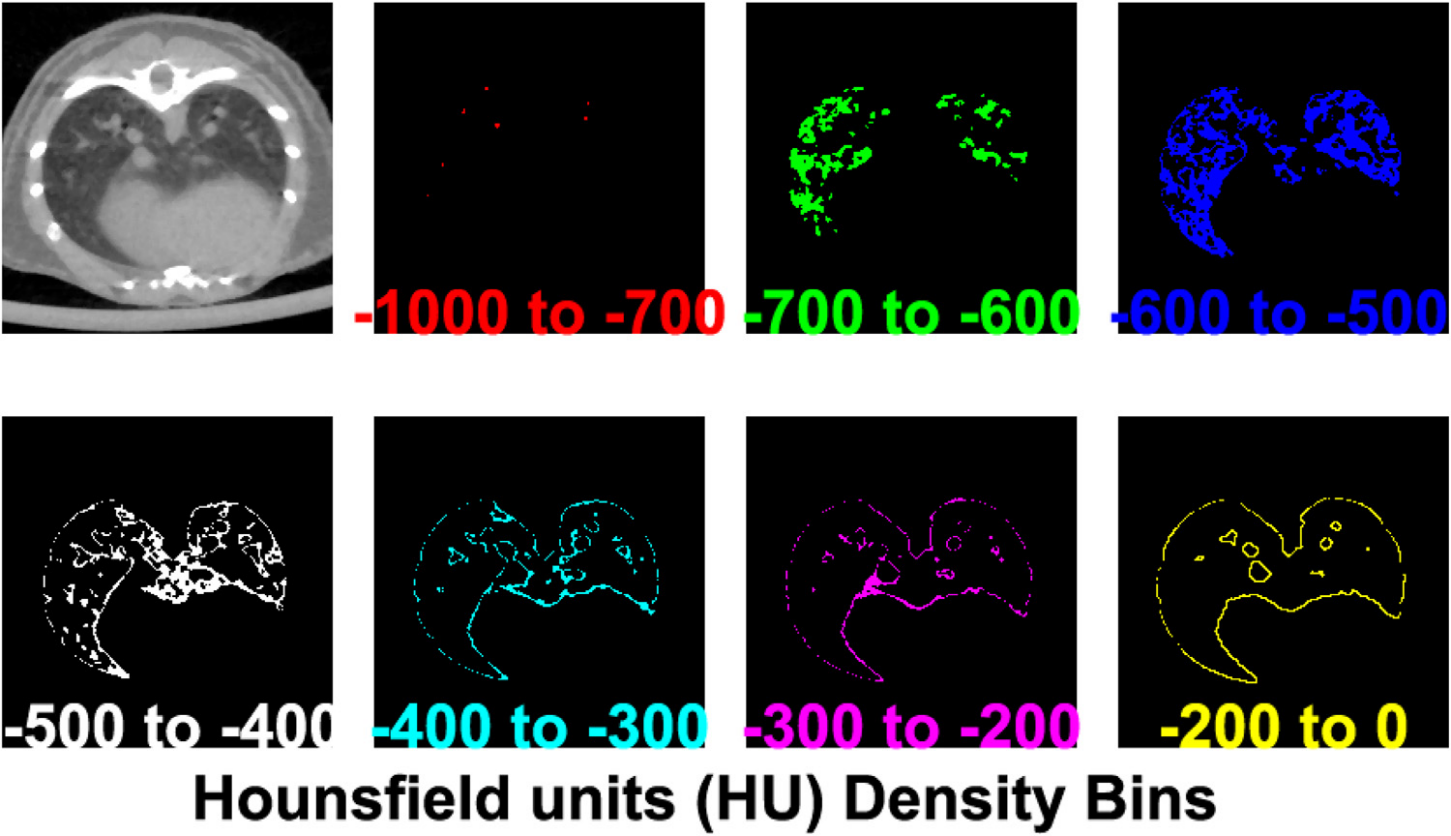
The Hounsfield Units (HU) that correspond to the lung tissue and connective tissue are color coded by intensity bin. Each bin range shows a unique portion of the lung morphology. Key bins are [−1000 to −700] corresponding to regions of air (e.g. lumen of large airways in this CT cross sectional slice), [−500] HU corresponding to areas having a 50/50 ratio of air/tissue, and [−200 to 0] for connective tissue.
